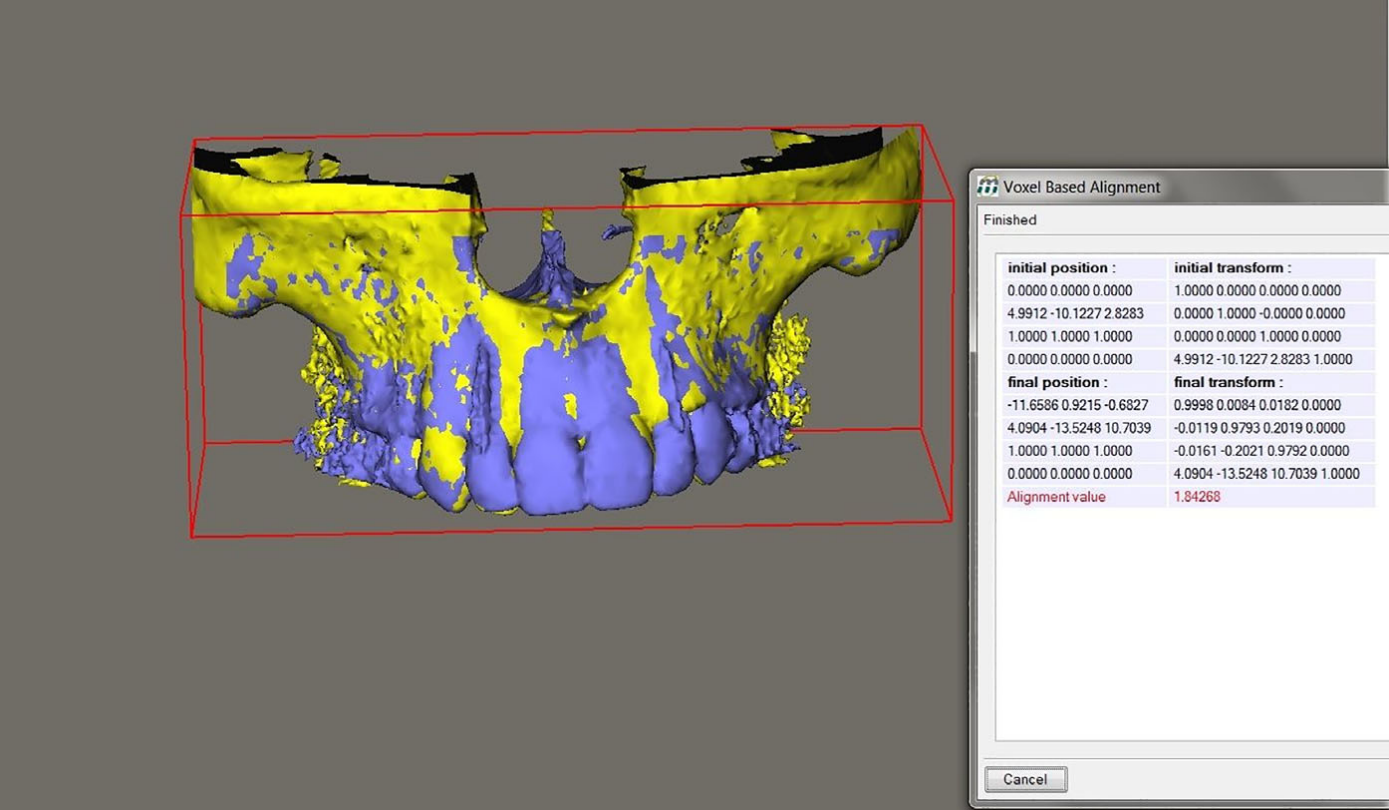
Alignment of images of pretreatment cone‐beam computed tomography (CBCT) and the 1‐month CBCT by the computing program Maxilim
